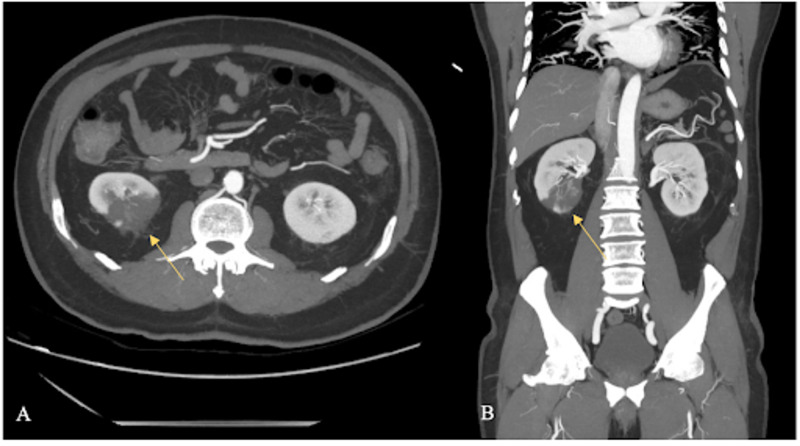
Axial image (A) and coronal image (B) from the arterial phase of a CTA demonstrating wedge-shaped regions of hypoenhancement within the lower pole of the right kidney. CTA, computed tomography angiogram
